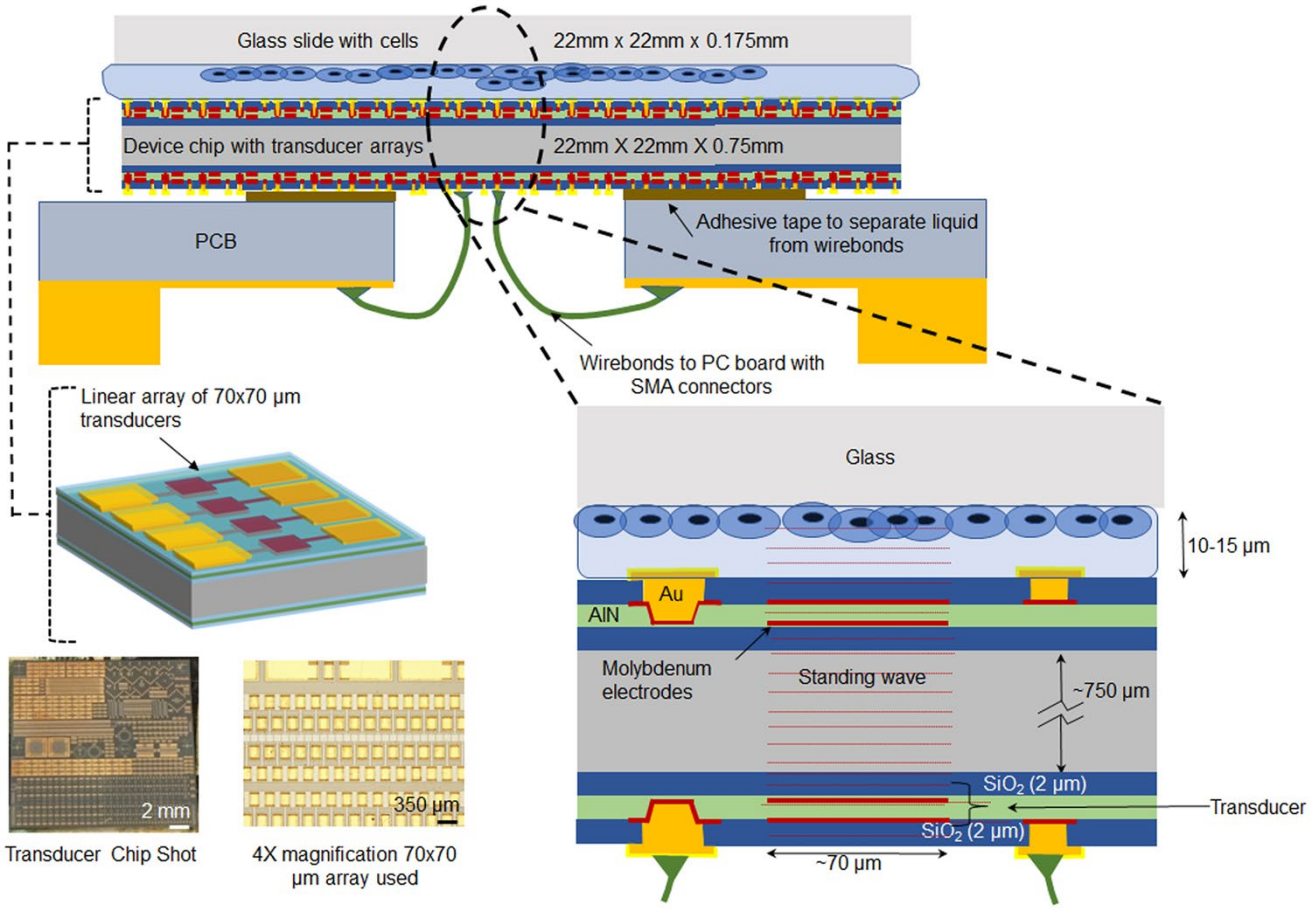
Sketch of ultrasonic transducer chip and packaging used for neural stimulation studies. (top) The AlN transducer chip is adhesively attached to an orifice in a PC board. Wire bonds are used to connect the transducer electrodes to PCB traces connected to SMA RF connectors. A glass cover slip with cell grown on the glass is placed on top of the chip with a thin media layer in between the glass and the chip. (bottom left) The silicon die is 22 mm × 22 mm with AlN transducers are either on one side or both sides of the chip. Gold electrodes on bond pads are used to connect to the PC board via wire bonds. Chip shot is taken after use with neurons, and no metal delamination or damage is observed. (bottom right) Waves generated from the bottom transducer setup a standing wave pattern through the die thickness affecting the neurons. Note that in the two-sided transducer silicon die, the electrodes for the top transducers are floating. The top transducers are aligned to the bottom transducers and are used to locate the cells in reference to the actuated transducers.
